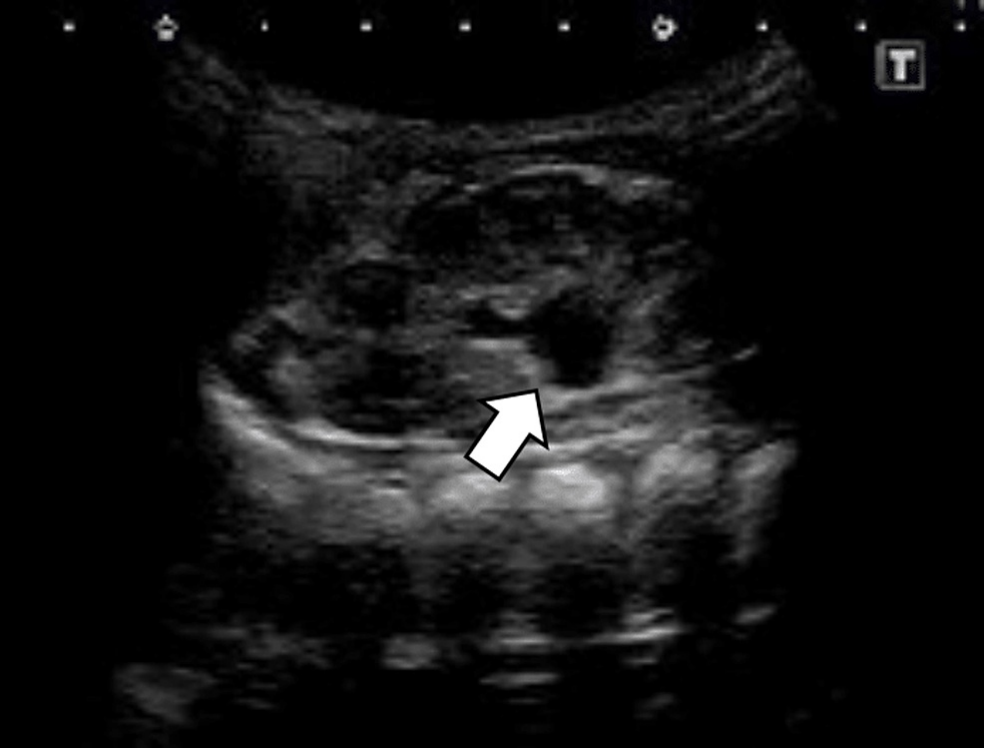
Ultrasound imaging Ultrasonography showing a mild expansion of the renal pelvis of the left kidney (arrow), which was classified as mild hydronephrosis based on the Society for Fetal Urology (SFU) grading system (grade 1)
